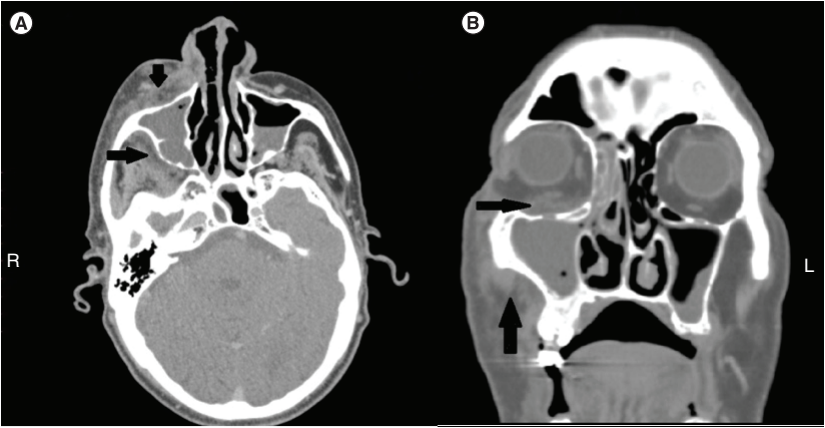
Head CT scan findings. (A) Axial head CT scan: necropurulent collection in the infraorbital (horizontal arrow) and infratemporal spaces (vertical arrow). (B) Coronal head CT scan: necropurulent collection in the orbital floor (horizontal arrow) and involvement of the buccal space (vertical arrow). Necropurulent collection in the right maxillary and ethmoidal sinuses.
